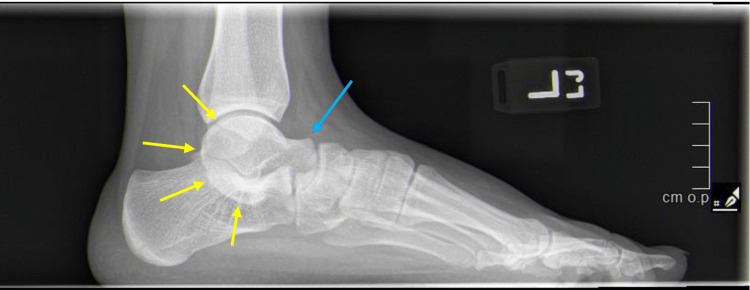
Lateral weightbearing radiograph of the left foot. Radiograph demonstrates signs of talo-calcaneal tarsal coalition including beaking of the talus (blue arrow) and “C-sign” (yellow arrows).
